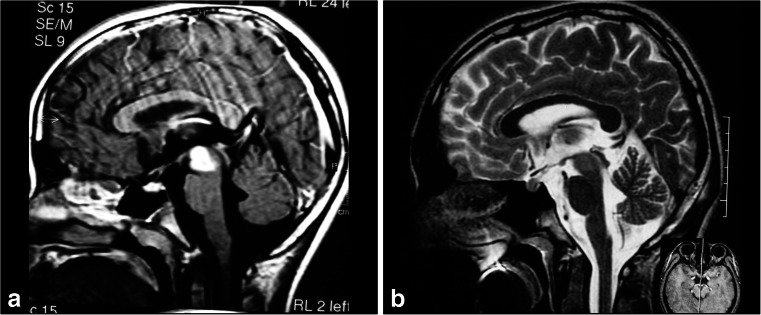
a. A 7-year-old patient with diplopia and paresis of the oculomotor nerve on the right side. MRI shows a focal and intrinsic lesion parallel to the intrinsic course of the third nerve in the midbrain. b. Follow-up 5 years after gross total resection showing no residual lesion
